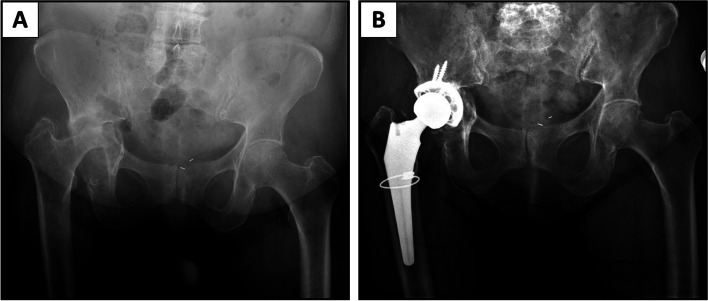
Complex primary THA: A Preoperative pelvis AP radiograph of 82 y/o female with previous acetabular fracture and arthrosis. B Postoperative AP pelvis radiograph of fully porous cup with cemented XLPE liner and two locking screws
